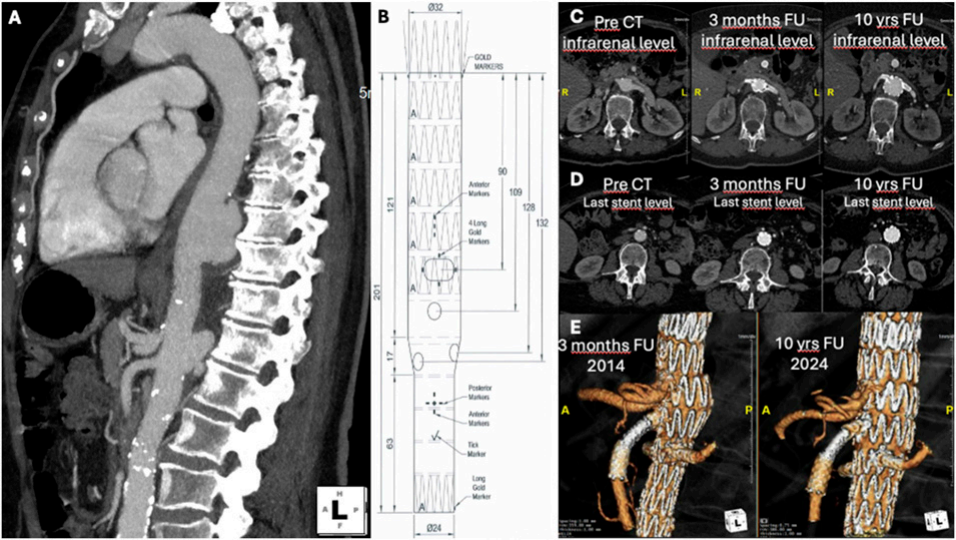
Case images. (A) Multi Intensity Projection (MIP) Computed Tomography Angiography (CTA) in sagittal view that shows the thoracic and visceral PAUs pseudoaneurysm; (B) fEVAR graft plan; (C-D) axial view of CTA at different time of the aortic level with the bigger diameter changes; (C) infrarenal level aortic diameter changes; (D) last stent level aortic diameter changes; (E) 3D reconstruction of the graft at 3 months follow-up (FU) and 10 years FU.
